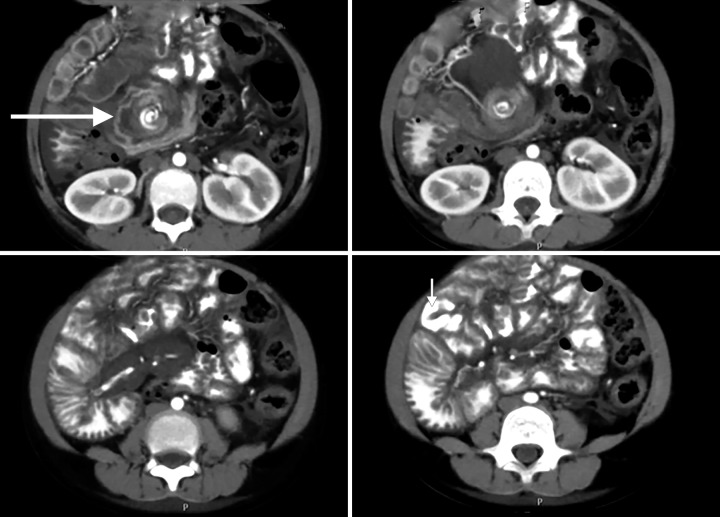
Contrast enhanced computed tomography shows duodenojejunal flexure on right side of spine with jejunal loops (filled with iodinated contrast predominantly on right side (small arrow). There is evidence of twisting of mesenteric vessels surrounding small bowel (whirlpool sign); midgut volvulus (large arrow)
